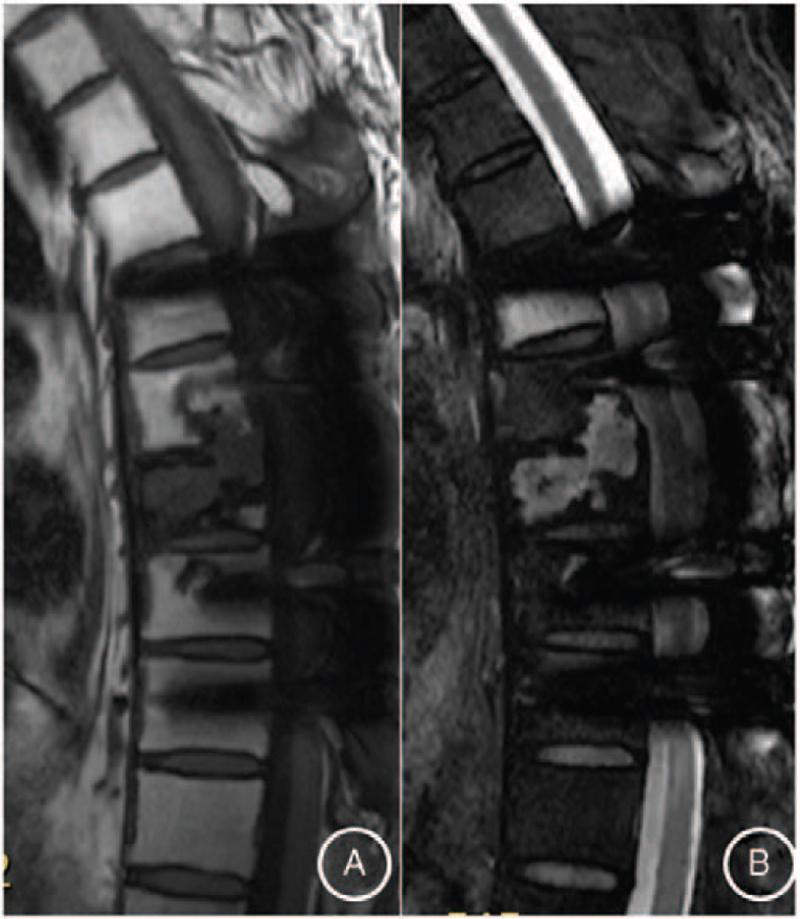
Thoracic spine magnetic resonance imaging at 6 months after radiotherapy showed the lesion was smaller compared to the pre-treatment imaging (A). The lesion exhibiting mildly isointense on T1 weighted image (B). Hyperintense on T2-weighted image.
